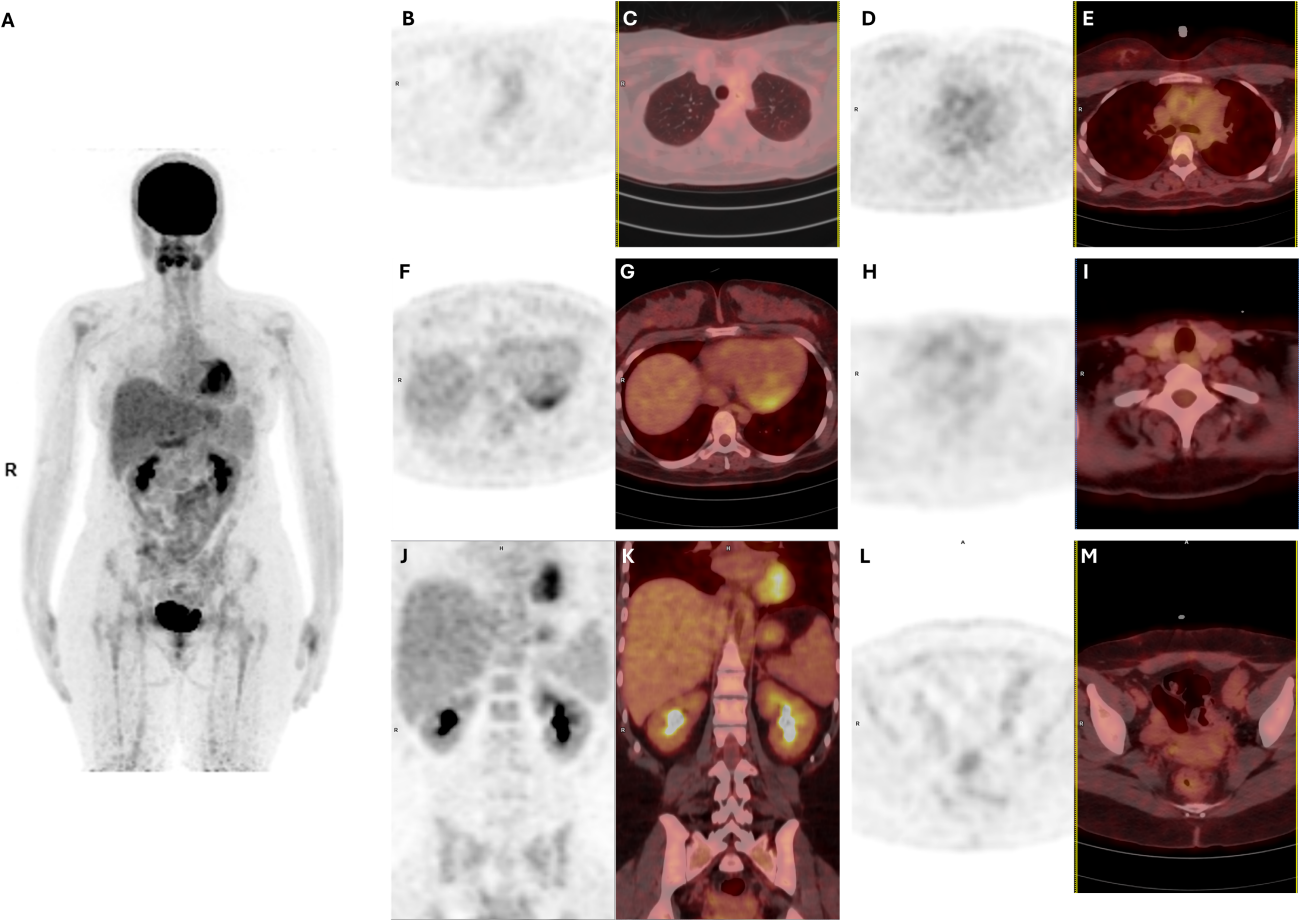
Post-treatment ^18F-FDG PET and fused PET/CT images demonstrating complete metabolic remission. (A) Whole-body PET MIP demonstrating resolution of previously observed FDG-avid extranodal disease. (B, C) PET and corresponding fused PET/CT images showing complete resolution of previously hypermetabolic pulmonary lesions. (D, E) PET and corresponding fused PET/CT images demonstrating absence of abnormal supradiaphragmatic FDG uptake. (F, G) PET and corresponding fused PET/CT images showing complete resolution of myocardial FDG uptake. (H, I) PET and corresponding fused PET/CT images demonstrating normalization of thyroid gland FDG uptake. (J, K) PET and corresponding fused PET/CT images demonstrating normalization of FDG uptake in the kidneys and adrenal glands. (L, M) PET and corresponding fused PET/CT images showing resolution of previously increased FDG uptake in the genitourinary organs.
